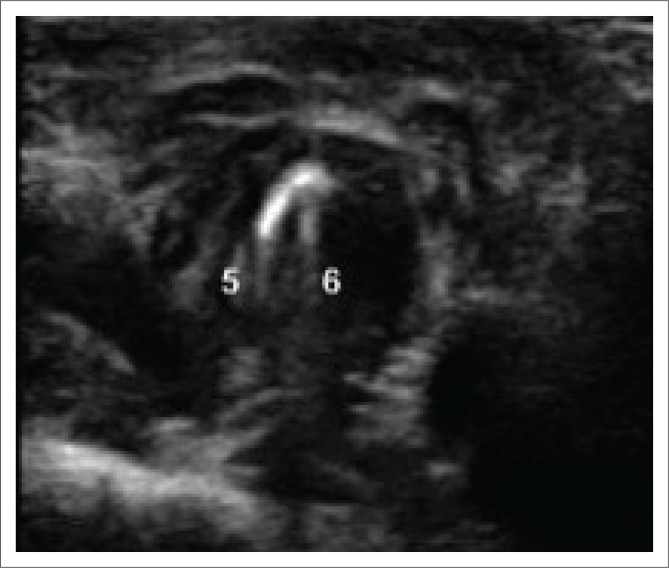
Translaryngeal ultrasonography of left vocal cord palsy where the following anatomical structures are visualised and numbered. (5) Right true vocal cord. (6) Left true vocal cord (fixed in midline).
